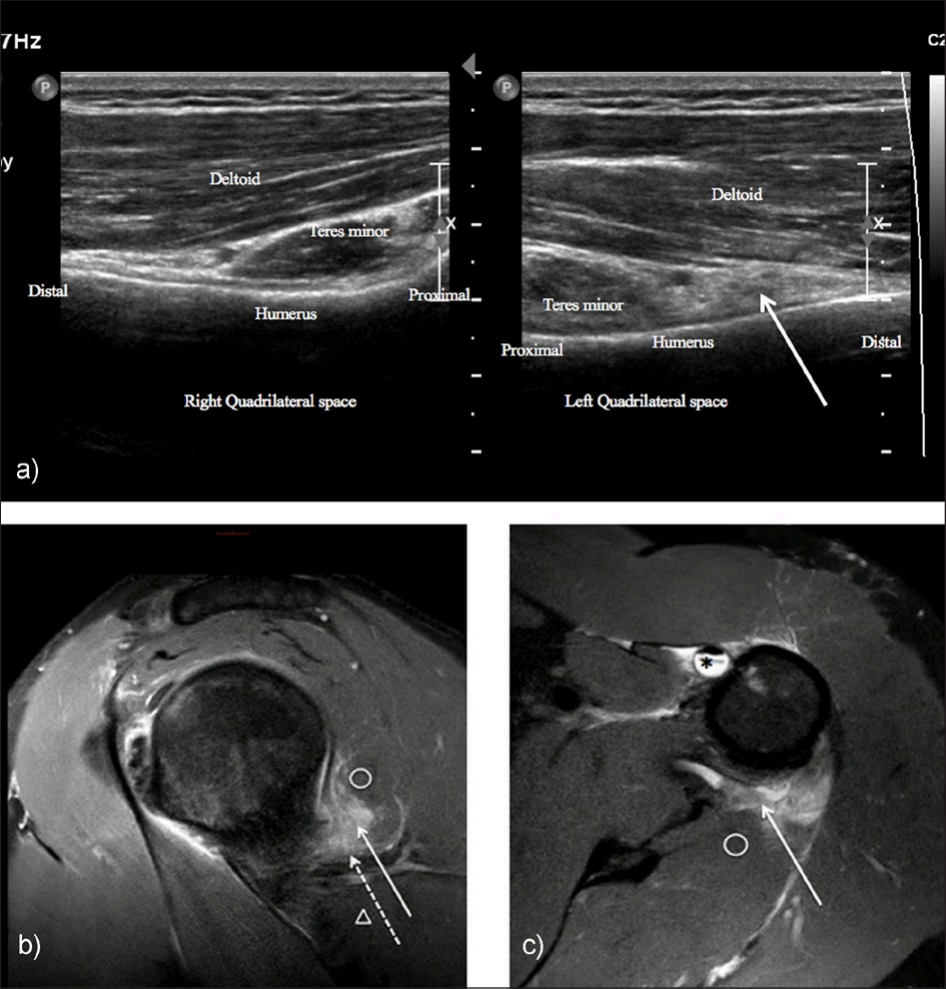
Neuropathy of axillary nerve after skiboard fall leading to injury of the shoulder capsule. Ultrasound showed infiltration of the axillary nerve (dashed arrow) in quadrilateral space (solid arrow) compared to normal side (4a = FIGURE 10 uploaded online manuscript), confirming by sagittal (4b = FIGURE 11 uploaded online manuscript), axial (4c = FIGURE 12 uploaded online manuscript) Proton Density with Fat Saturation MRI images. Note the concomitant Biceps brachii tendon injury (star). Teres minor muscle (circle) – Teres major muscle (arrowhead).
